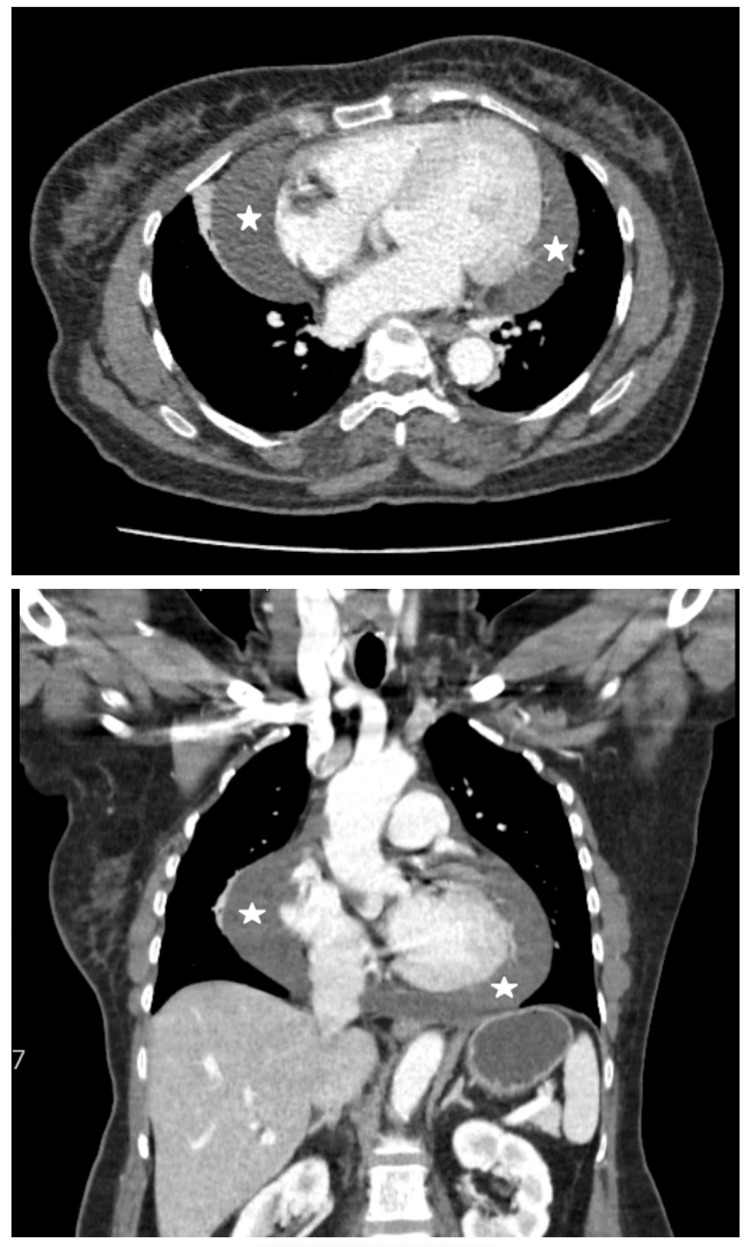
CT of the thorax revealing a large pericardial effusion, as depicted by the white star
